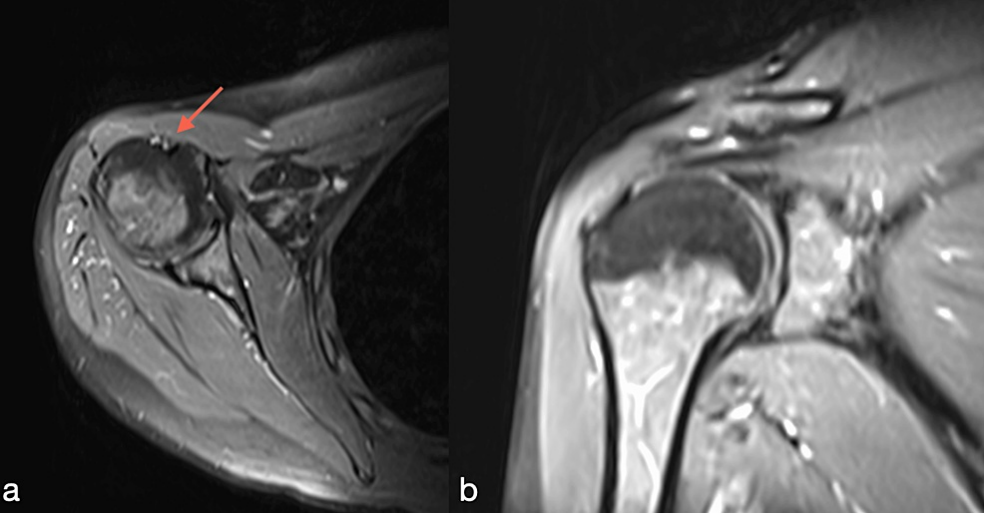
Magnetic resonance imaging of (a) the axial shoulder, showing biceps tendinopathy (arrow) and (b) the coronal shoulder showing no concominant rotator cuff pathology.
